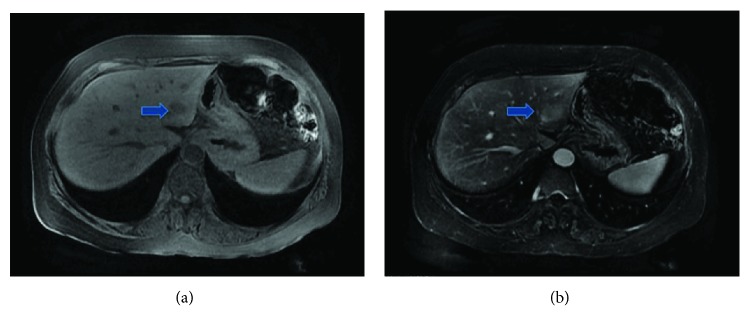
Magnetic resonance imaging (MRI) axial cuts showing the left lateral section liver lesion being hypointense on T1-weighted images in (a, blue arrow) and slightly hyperintense on T2-weighted MRI images in (b, blue arrow).
